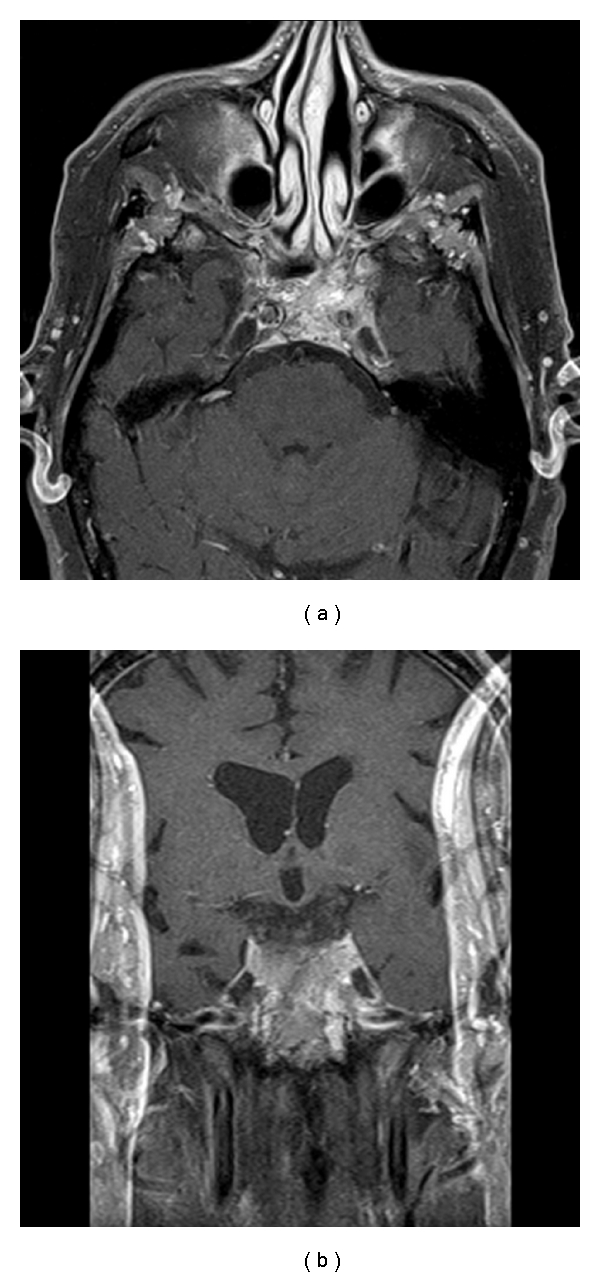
Axial and coronal T1-weighted MR imaging with gadolinium enhancement showing a homogenously enhancing soft tissue mass involving the clivus, pituitary fossa, cavernous sinus, and posterior nasal cavity.
